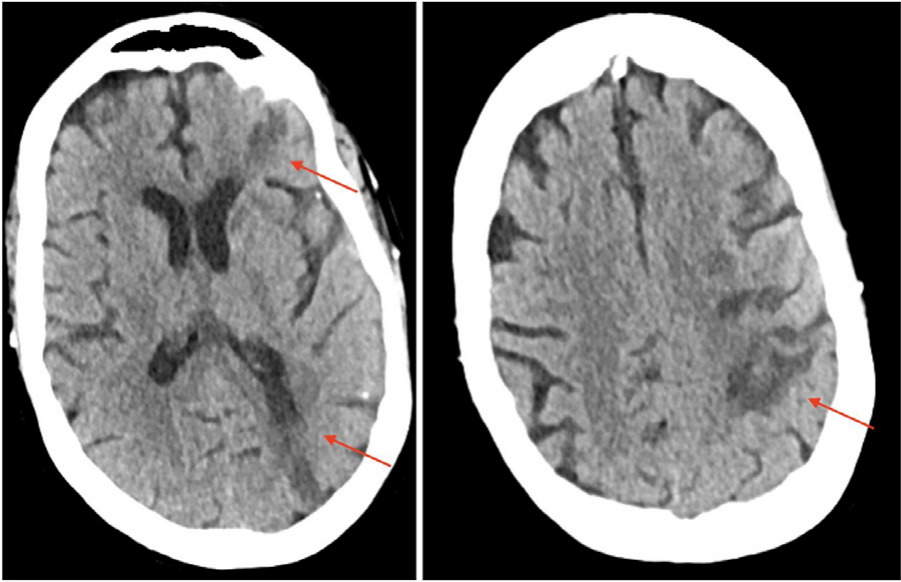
9 days post admission, axial CT heads without contrast demonstrating expected evolution of multiple left hemispheric acute/subacute infarcts. Image on left demonstrates left-sided temporo-occipital and frontal infarcts, while the right image demonstrates temporo-occipital infarct.
